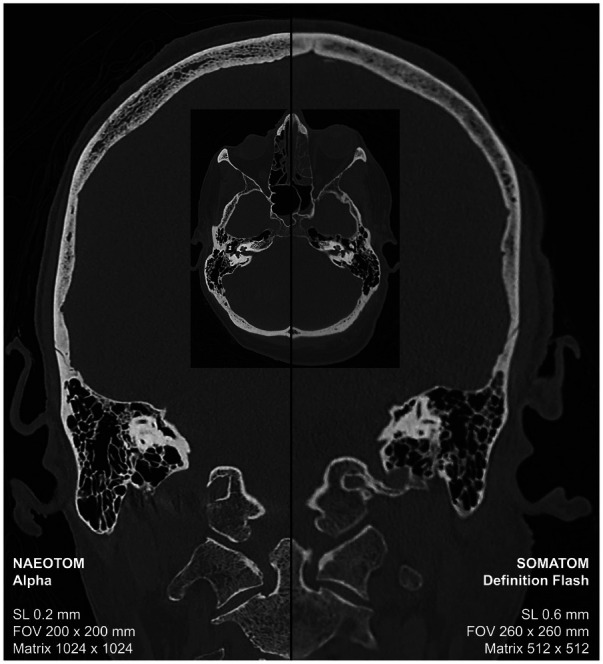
Postmortem CT scan for the virtual autopsy of a deceased person using photon-counting CT (left-sided) and conventional CT with energy-integrating detectors.
